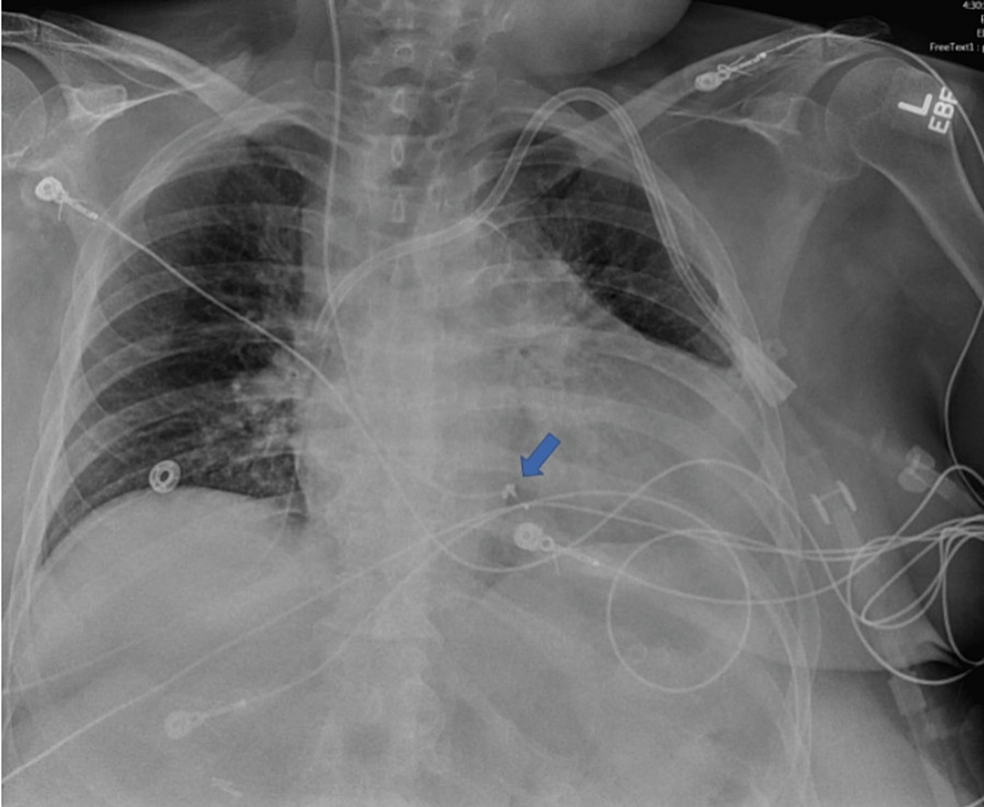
A chest radiograph with an arrow showing a distal knot in the pacemaker lead The image shows an anteroposterior radiograph with a left subclavian vas-cath. The right internal jugular temporary transcutaneous pacemaker with a distal knot is highlighted by the blue arrow.
